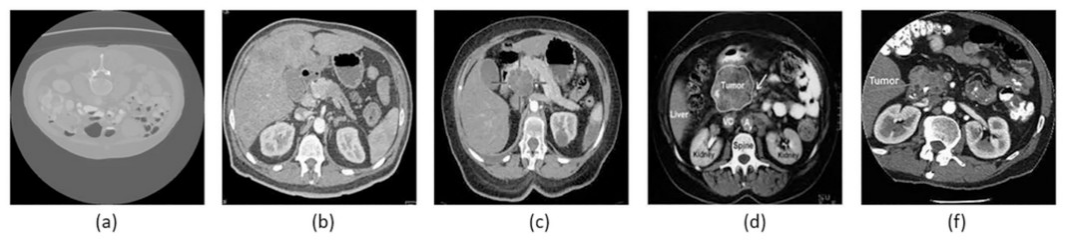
Dataset of CT images for pancreatic cancer.
